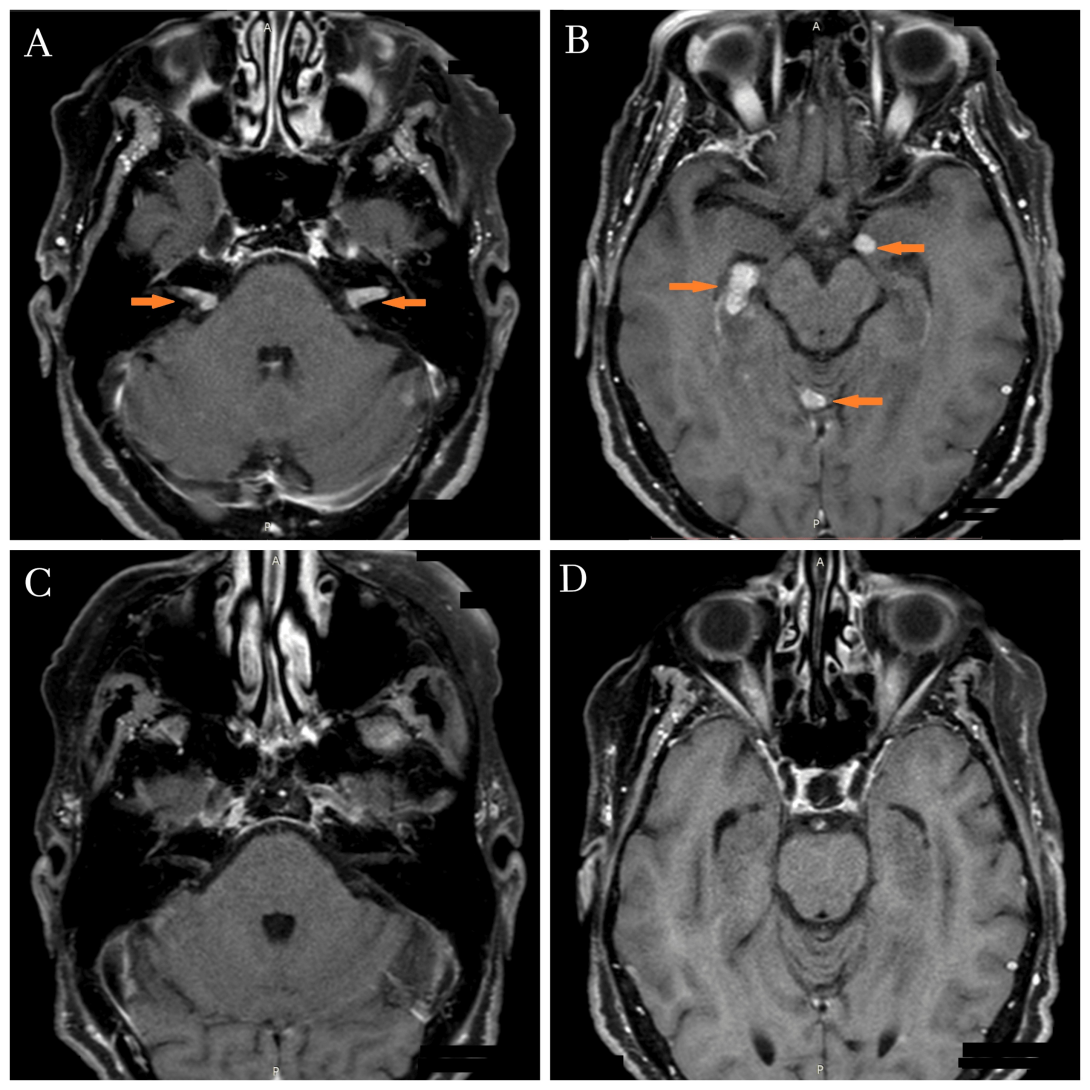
Cerebral magnetic resonance imaging (MRI) performed before and after the initiation of treatment with selpercatinib. Gadolinium-enhanced cerebral MRI revealed an enhancing lesion within the internal auditory canal (arrows) (A) and bilateral expansile lesions in the cerebellar hemispheres (arrows) (B), measuring less than 15 mm. At the three-month follow-up after initiating selpercatinib, MRI revealed near-complete resolution of the metastatic lesions, demonstrated by the minimal gadolinium enhancement in comparison to the baseline (C and D).
